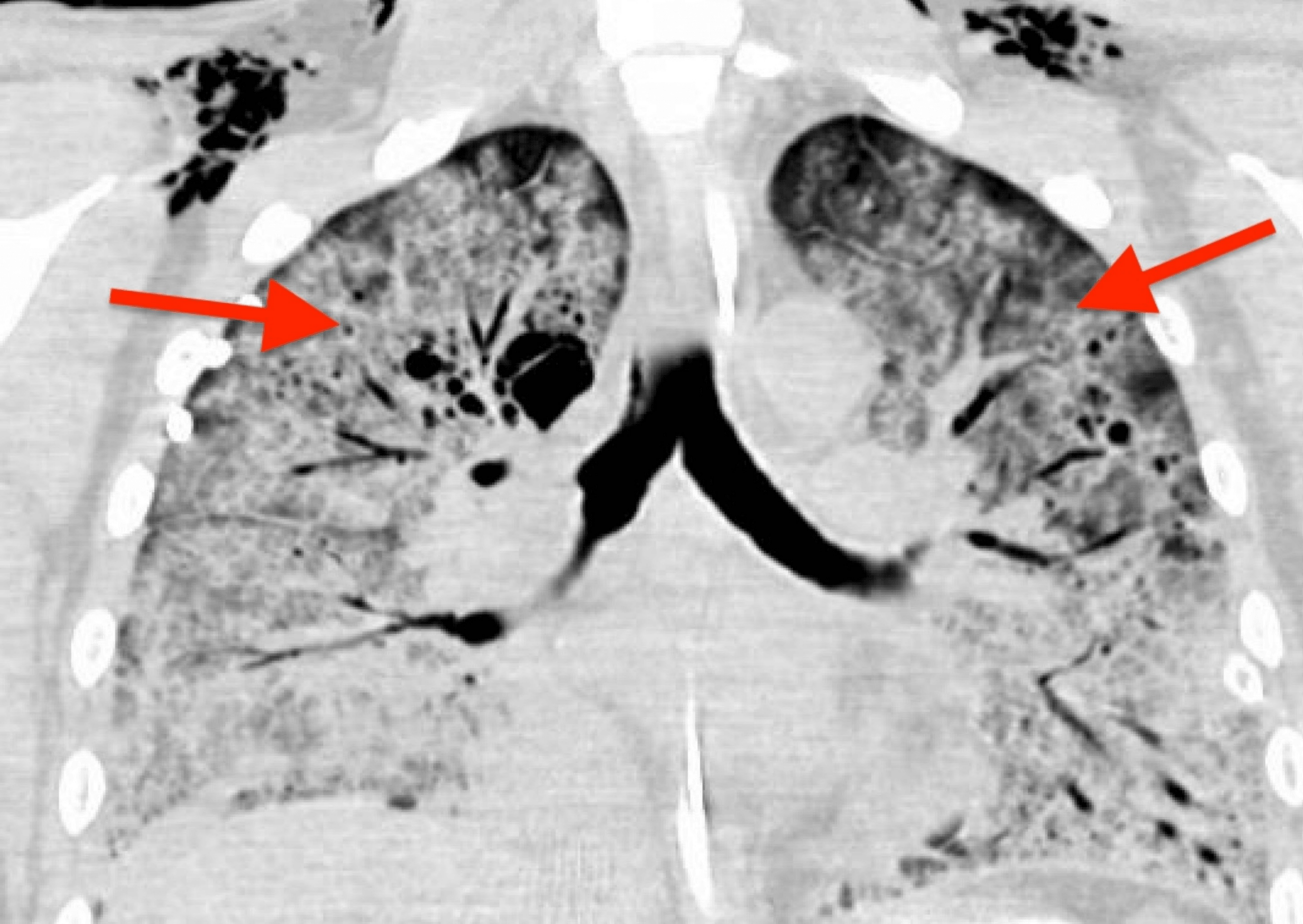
Coronal CT of the chest with contrast demonstrating bilateral ground glass opacities.
